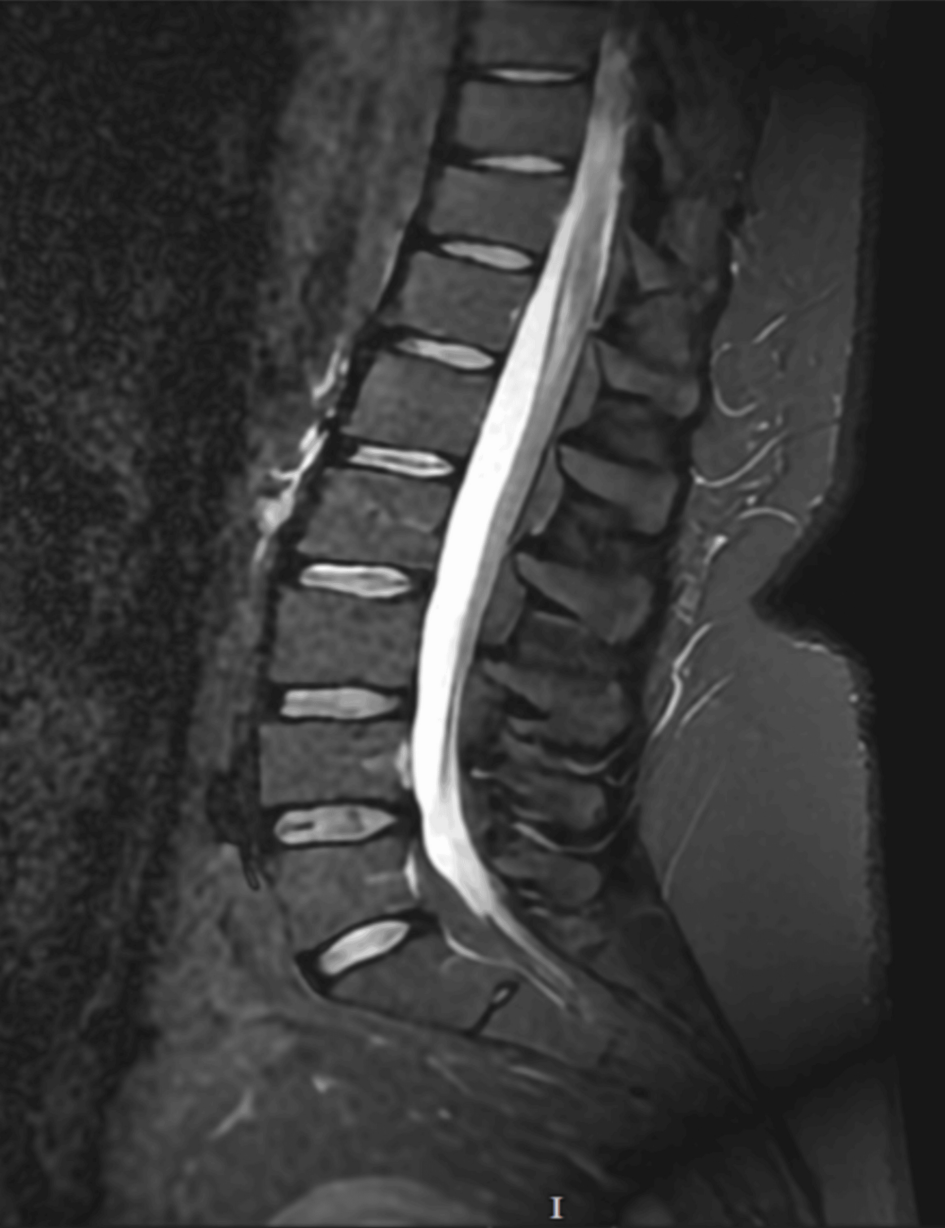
MRI of Lumbosacral Spine Showing Mild Diffuse Disc Bulging of L4/L5 Without Significant Neural Compression
